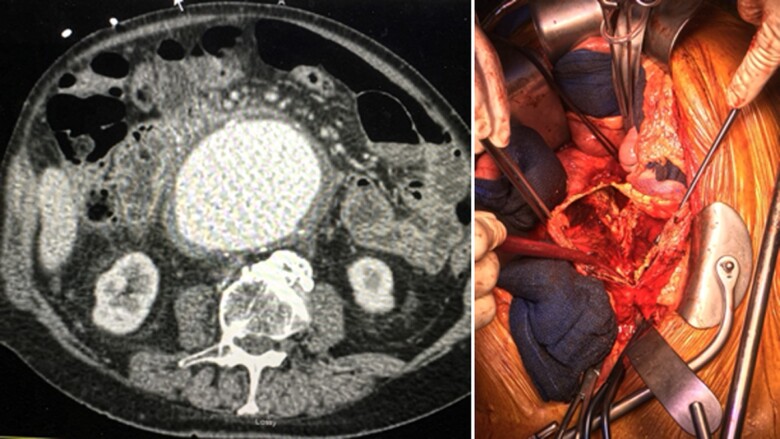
Computed tomography angiography transaxial image (left) and intraoperative (right) findings of a patients with a large abdominal aortic aneurysm.
